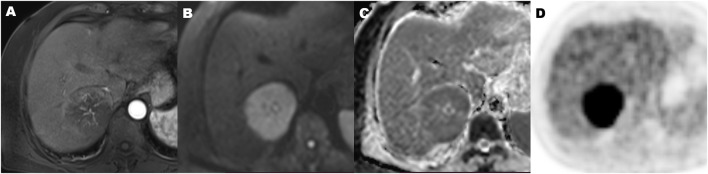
A 70-year-old hepatocellular carcinoma in liver segment VII woman with microvascular invasion, demonstrating Hypovascular enhancement pattern, high metabolic of SUVmax 9.2, MTV 134.2 cm^3^, TLG 915.2, TLR 4.5, and ADC of 0.86 × 10^−3^ mm^2^/s. Contrast-enhanced MR in arterial phase image (A), diffusion-weighted MR image (B), ADC map (C), and ^18^F-FDG PET (D) (MTV, metabolic tumor volume; TLG, total lesion glycolysis; TLR, ratio of tumor-to-liver SUV; ADC, apparent diffusion coefficient).
